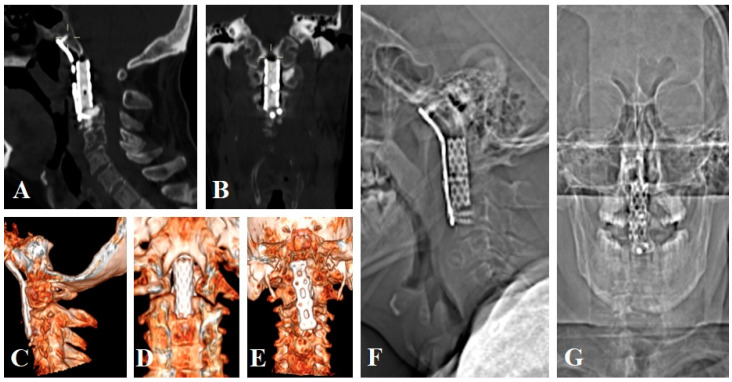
Postoperative CT scans (A,B), 3D reconstructions (C–E), and lateral (F) and anterior (G) x-rays show the clivus-cervical C3 stabilization and vertebrocementoplasty of the left lateral mass of C1 and the body of C3.
